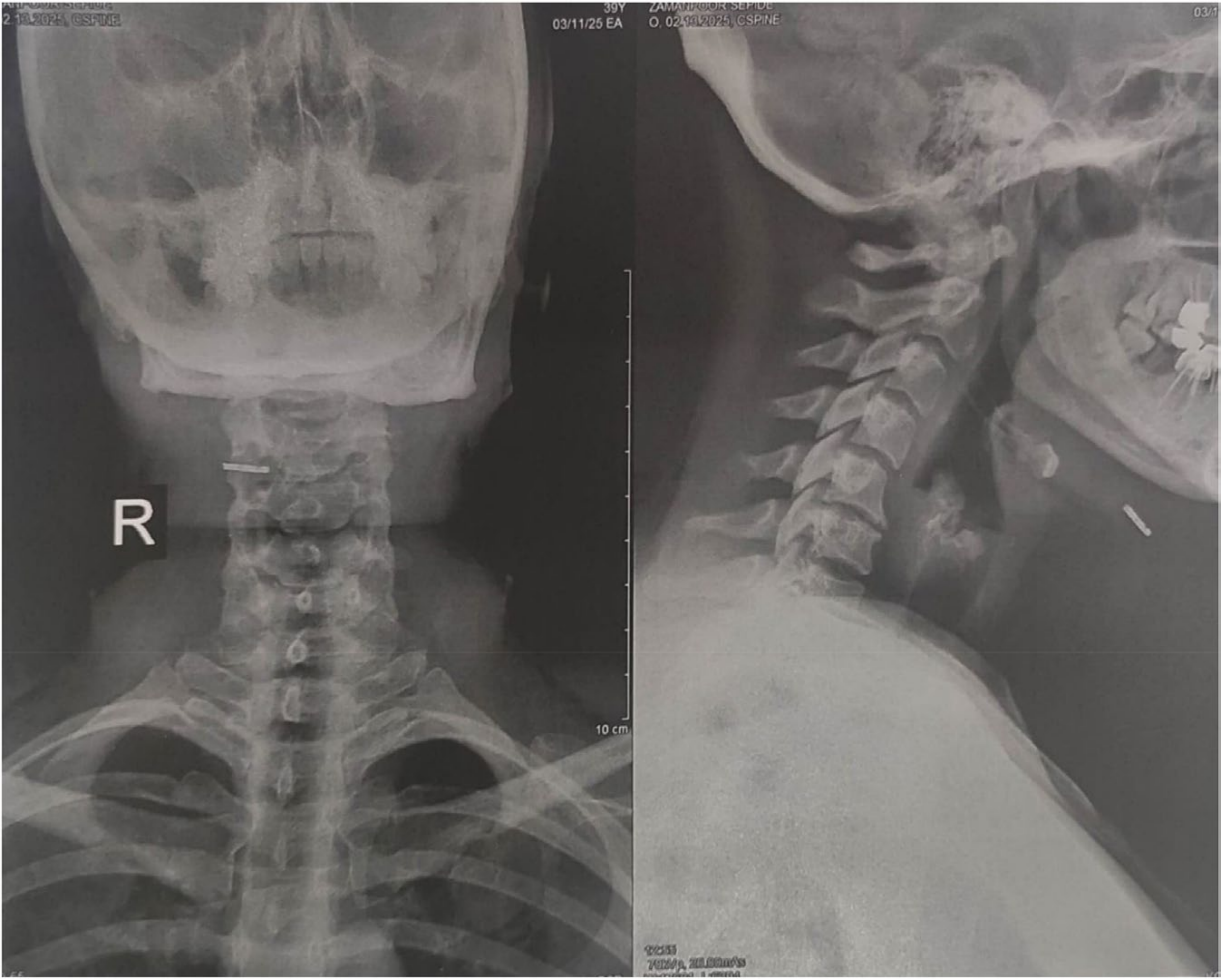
Neck radiography of a 42‐year‐old woman with a broken cannula tip embedded underneath her chin. The broken tip is visible anterior to the hyoid bone. (Left) Antero‐posterior view. (Right) Lateral view.
